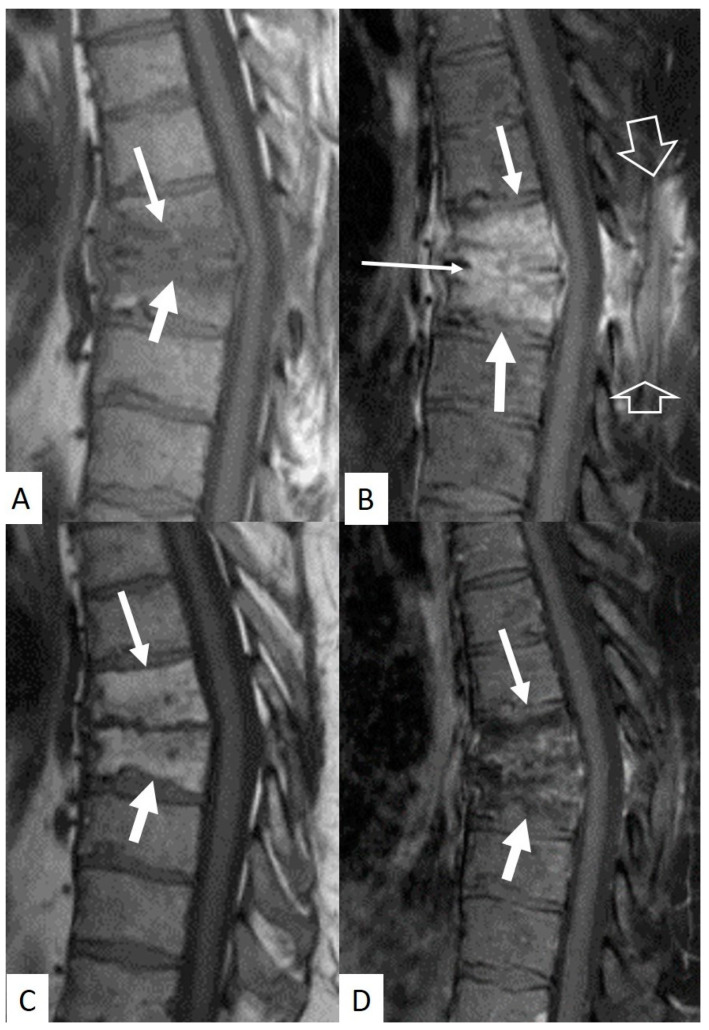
A 47-year-old male patient with *Salmonella* spondylodiscitis following previous discectomy and hemilaminectomy, 8w prior to imaging. (A) Sagittal T1-w MR image, showing bone marrow edema (arrows). (B) Sagittal fat suppressed contrast-enhanced MR image showing enhancement of the bone marrow lesions (arrows), enhancement of the intervertebral disc T7–T8 (horizontal thin arrow), mild kyphosis and normal postoperative changes posteriorly (open arrows). Sagittal T1-w (C) and fat suppressed contrast-enhanced T1-w (D) MR images 20 weeks after initiation of antibiotic treatment, show fatty metaplasia in the bone marrow of the T7 and T8 vertebral bodies without any abnormal enhancement, in keeping with healing (arrows). Note resolution of the postoperative findings.
